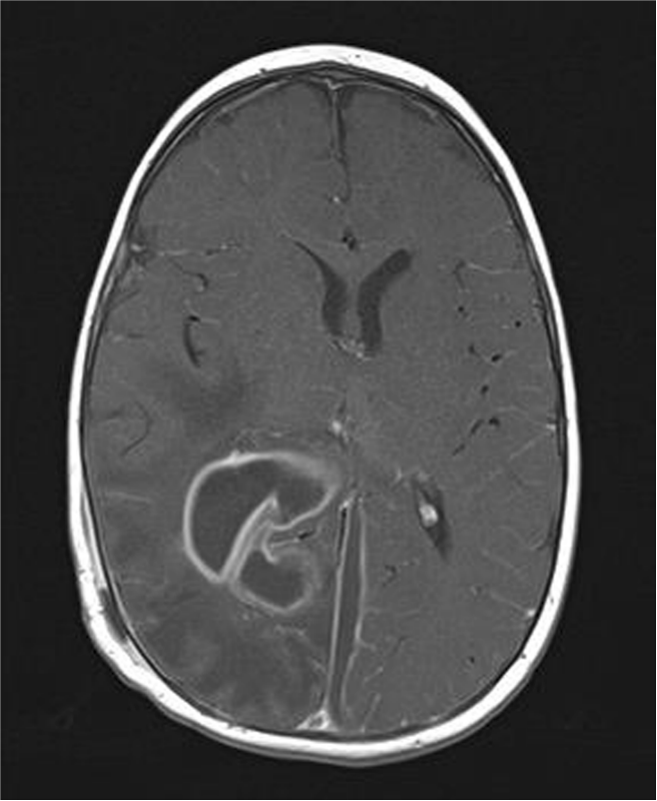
Initial post-op magnetic resonance imaging.
